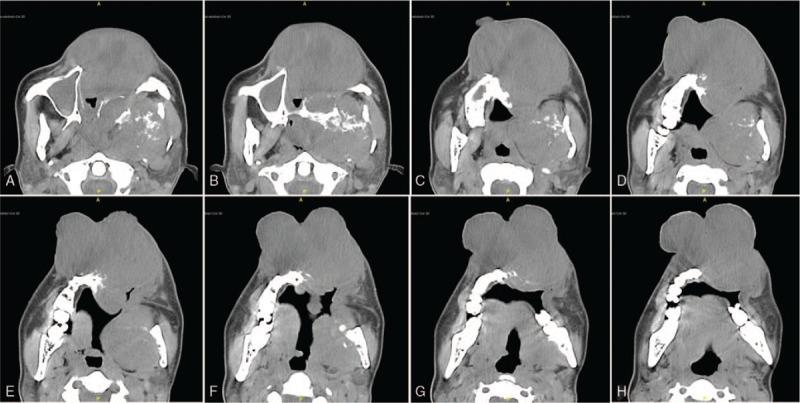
(A–H) Skull transverse CT scan revealed giant occupying lesions with extranasal involvement.
